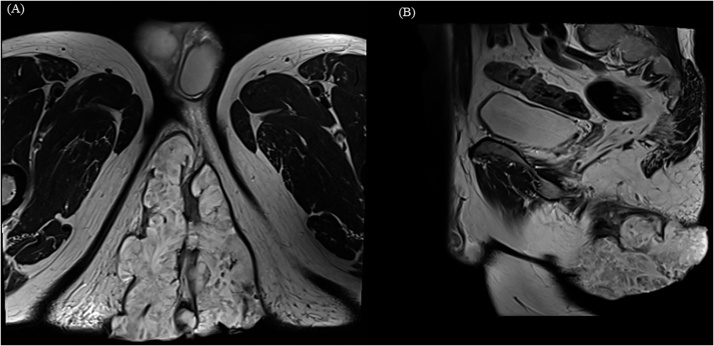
MRI findings. MRI showed 12 × 11 cm sized huge mass with extension to external anal sphincter and levator ani muscle. There was no evidence of lymphadenopathy in perirectal space and both pelvic wall. (a) T2 weighted Magnetic resonance imaging. Axial view. (b) T2 weighted Magnetic resonance imaging. Sagittal view.
